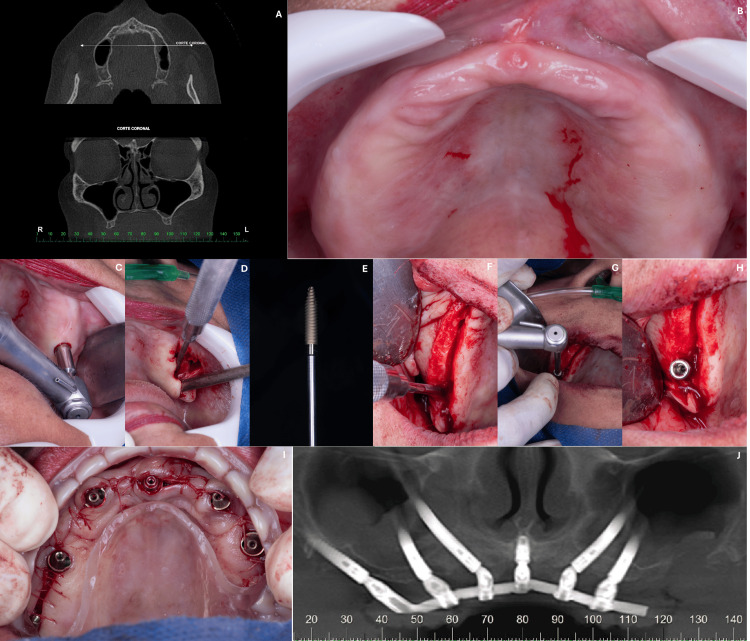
(A, B) Clinical and radiographic assessments revealed a severely atrophic maxilla associated with enlarged maxillary sinuses; (C-H) the treatment plan included the placement of six implants to support a maxillary full-arch prosthesis: two pterygoid implants, three zygomatic implants placed posteriorly, and one conventional implant; (J) the prosthesis was installed and supported by all implants and has been in function for 13 months without complications
